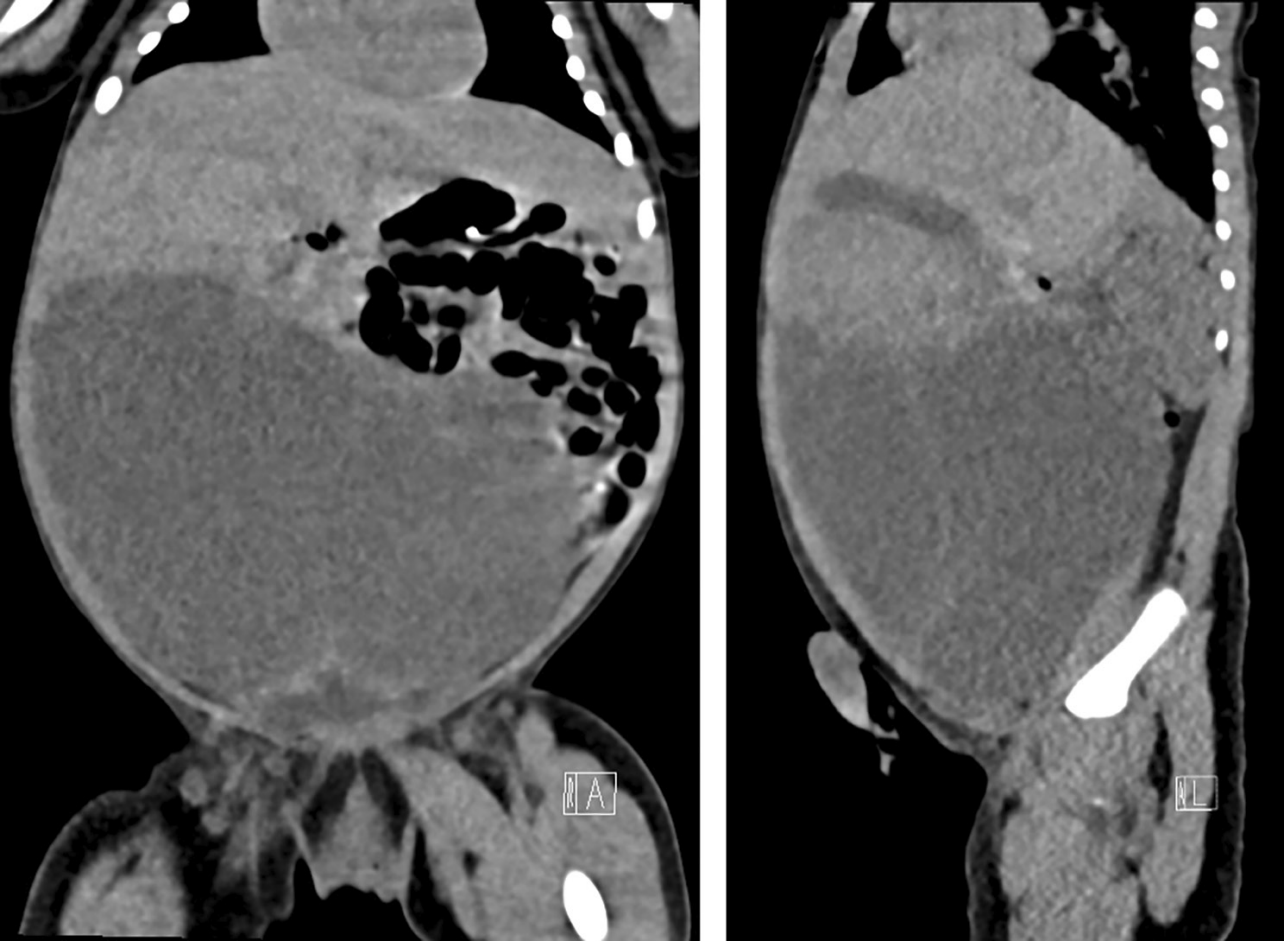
Coronal and sagittal computed tomography imaging showing a large cyst lesion of unknown origin, with a smooth outline. Thin septa are seen extending within the lesion. Attenuation values suggest serous fluid, being approximately +20 HU. No signs of hemorrhage or calcification seen.
